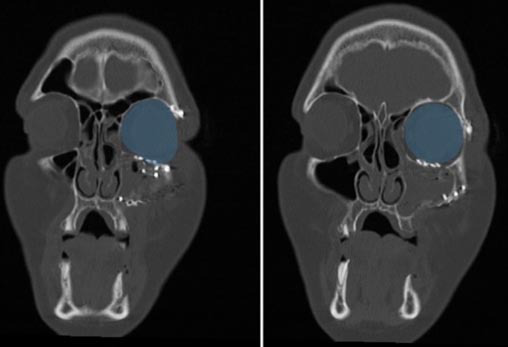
Postoperative computed tomography (CT) views of the reconstructed left orbit in the coronal plane
